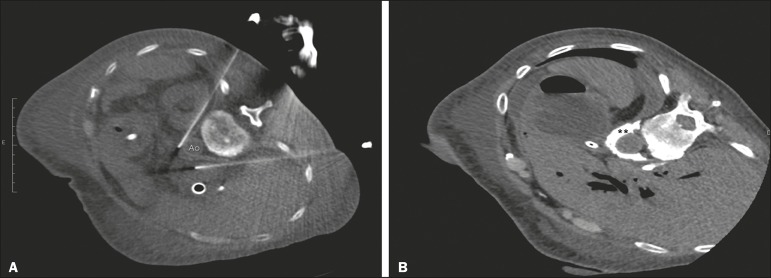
A: Axial CT scan, with the patient in the supine oblique position, showing bilateral paravertebral posterior punctures, adjacent to the aorta (Ao), at the level of the celiac trunk, made with a 22G Chiba needle. B: Correct distribution of absolute alcohol solution and iodinated contrast (asterisks).
